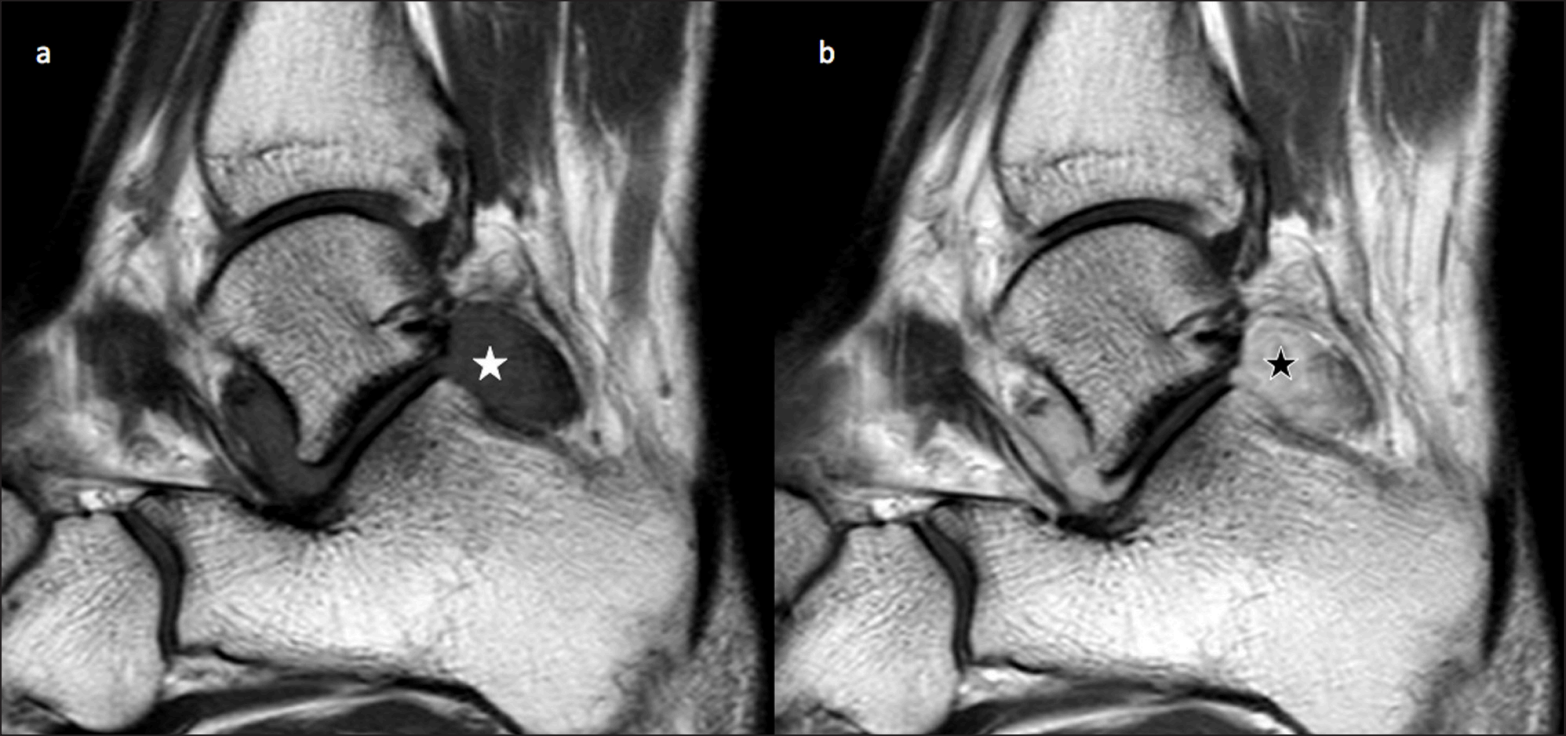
a. Sagittal T1-weighted image without gadolinium injection shows an intra-articular hypointense mass (white star) in the subtalar joint. b. Sagittal T1-weighted image with gadolinium injection shows the mass with heterogeneous enhancement (black star).
